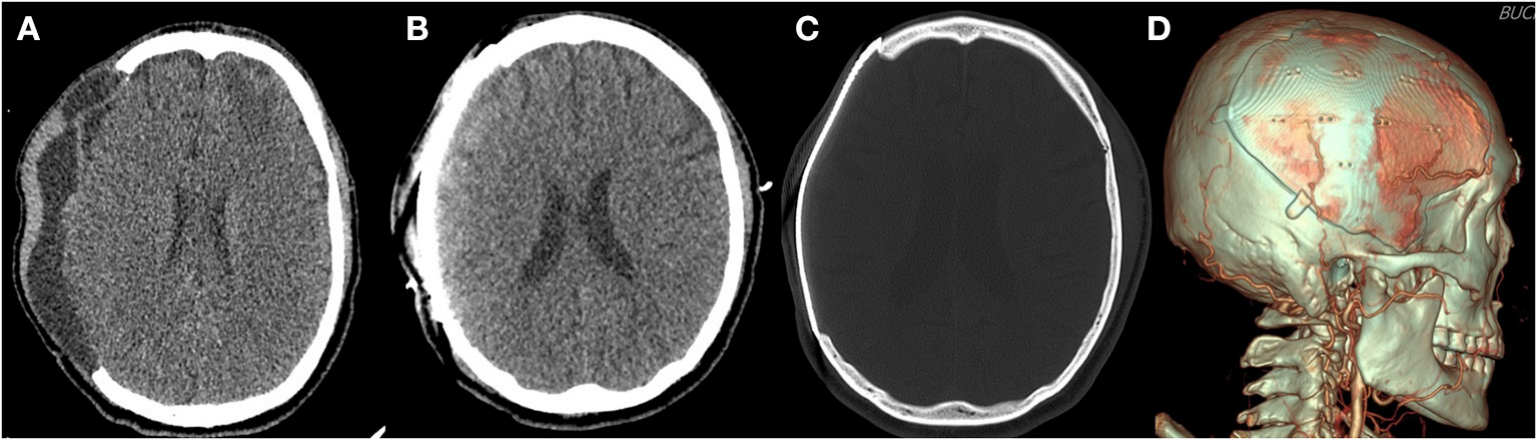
Three-dimensional (3D) printed patient-specific titanium implant. (A) The preoperative computed tomography scan of a patient with subgaleal and epidural fluid collection before cranioplasty. (B) The immediate postoperative computed tomography scan reveals no postoperative fluid collection after surgical evacuation during cranioplasty. (C) Bone setting view. (D) 3D reconstructed view.
